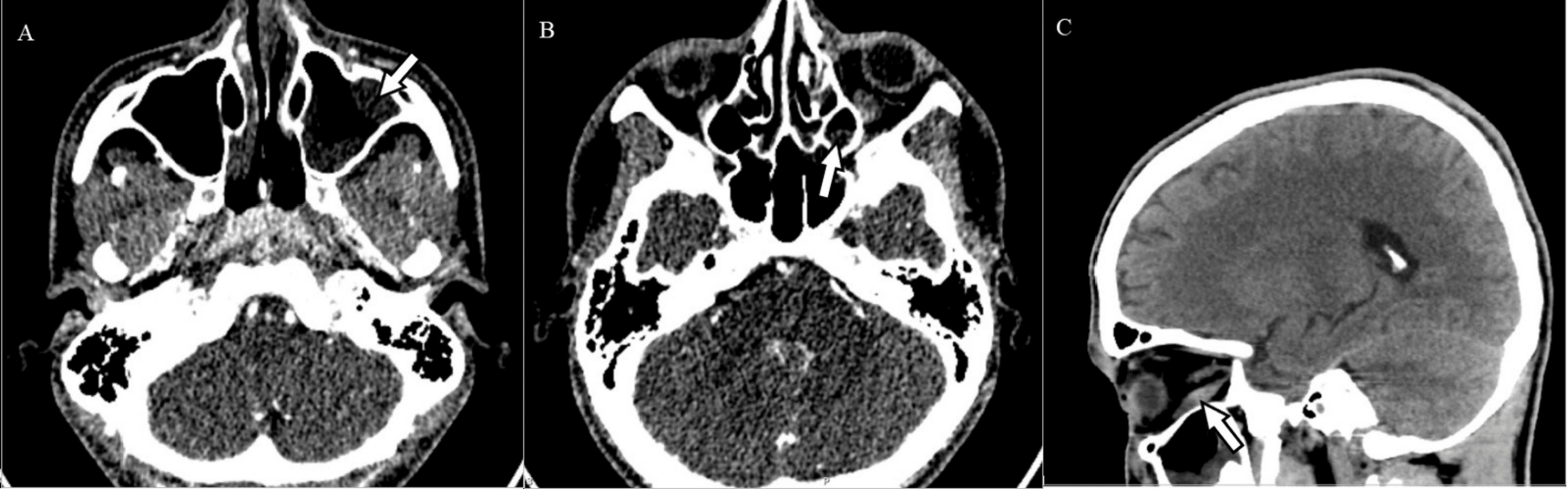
Head computed tomography scan at presentation The arrow shows (A) diffuse inflammation of left maxillary sinus mucosa, (B) inflammation of ethmoid sinus mucosa, and (C) diffuse densification of left intraorbital adipose tissue and increased thickness of homolateral inferior rectus muscle. All signs indicate an active rhino-orbital infection.
